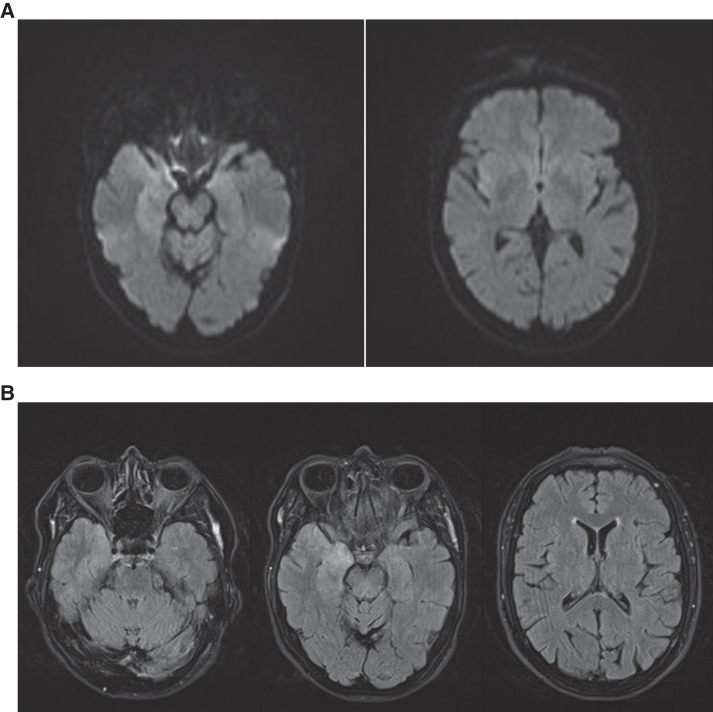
Brain magnetic resonance imaging (MRI) on day 2. (A) Brain MRI, DWI: hyperintense signals in the right inferior temporal gyrus and juxta insular. (B) Brain MRI, FLAIR: hyperintense signals in the same parts of the DWI sequences.
